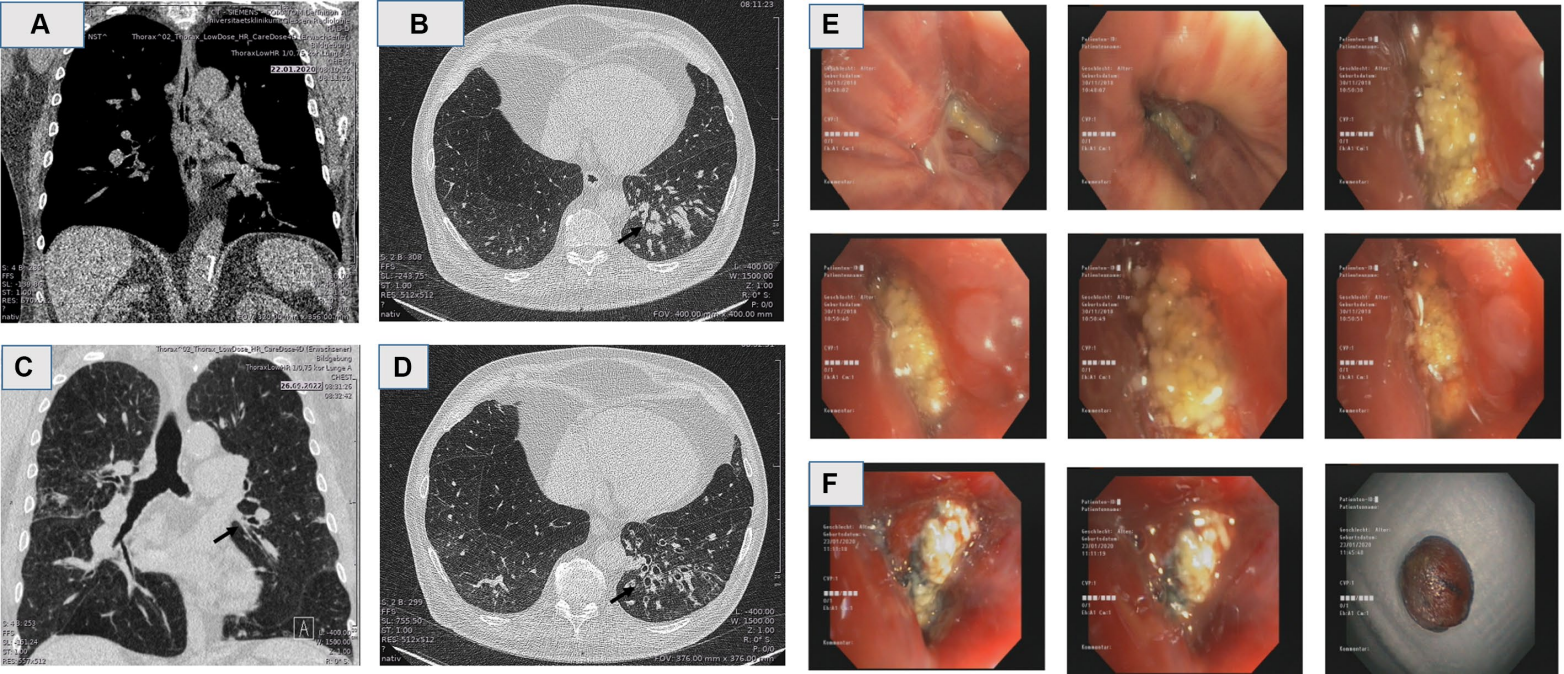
(A,B) Computerized tomography (CT) in coronal and axial reconstruction demonstrated compression of the lower left bronchus, followed by mucus obstruction of the bronchi, and post-stenotic bronchiectatic actinomycosis. (C,D) Computerized tomography in coronal and axial reconstruction in follow up, months post extraction of the cherry pit, revealing complete resolution with remaining bronchiectasia distally. (E,F) flexible bronchoscopy of the left lower lobe at initial presentation revealed an actinomycosis in biopsy (E), and remaining obstruction in 2 years follow up, with the macroscopic appearance of the aspirated material, mimicking lung tumor (F).
